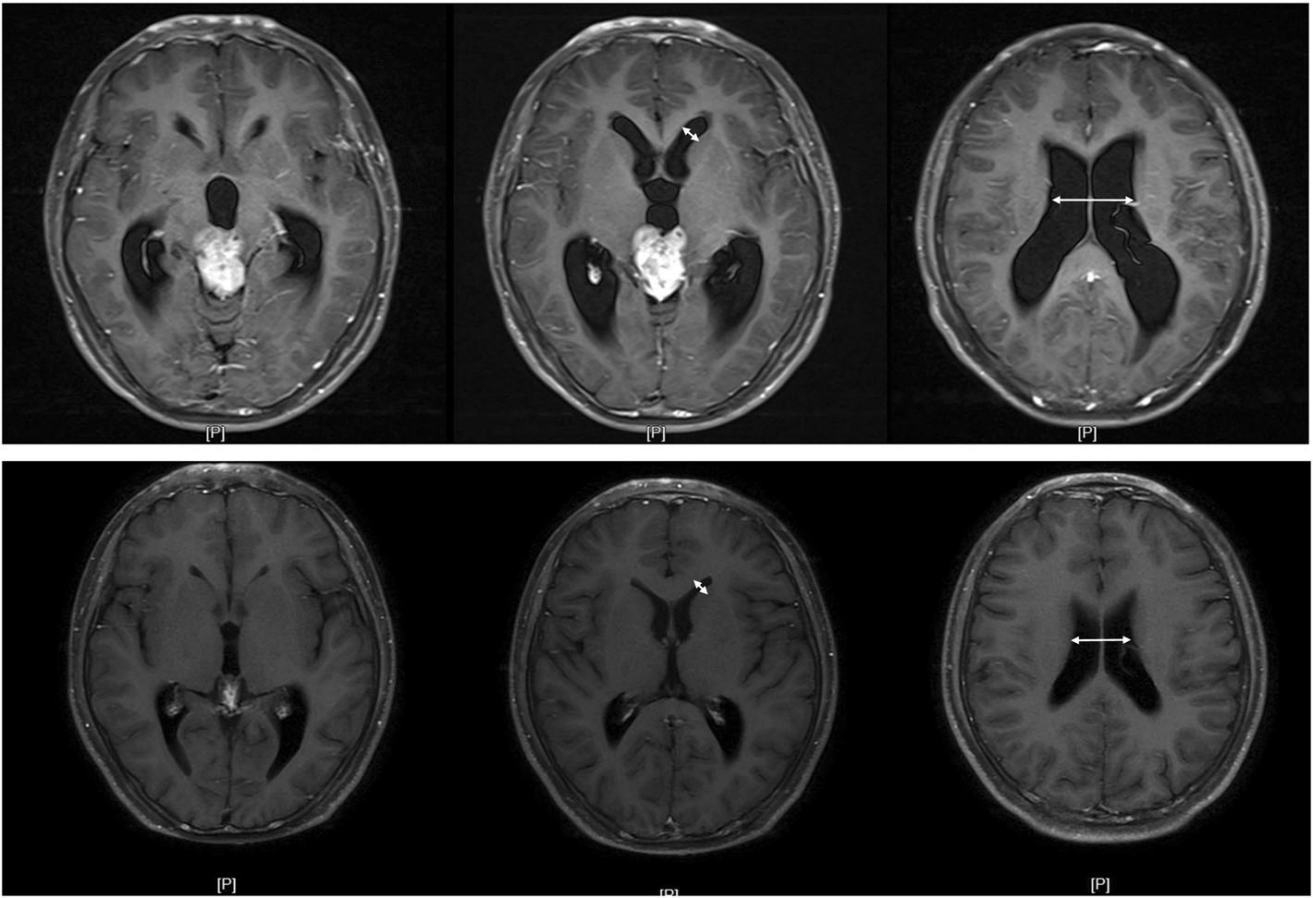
The maximum width of frontal horn (FH) and the minimum width of trunk of corpus callosum (TCC) (white arrows) on T1-weighted enhanced-contrast images were measured to evaluate hydrocephalus changing before (upper axial images) and after (lower axial images) emergency irradiation
